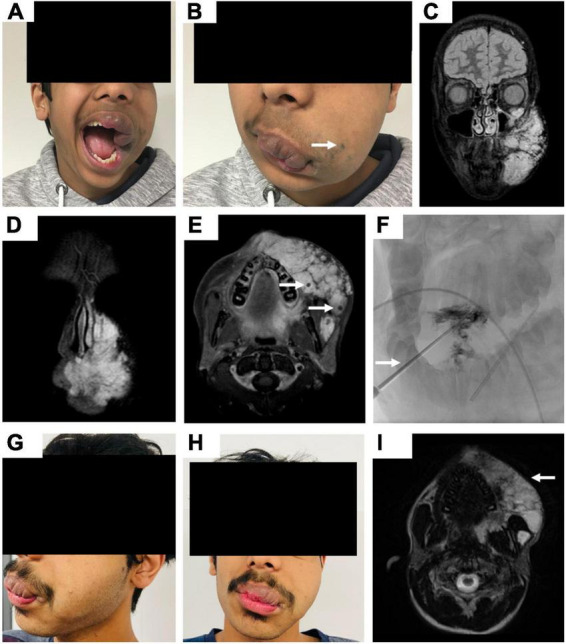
Fourteen-year-old male patient with a venous malformation (VM) primarily affecting the nasal, buccal, mental, and labial area of the left face. (A,B) Clinical photographs demonstrate the appearance of the patient with extensive swelling in the areas affected by the VM and enormous labial deformation on the left side. Superficial bluish discoloration of the skin is marked by an arrow. (C–E) Coronar and axial fat-saturated T2-weighted MR images present typical hyperintense signal of the facial VM with multiple hypointense spots representing phleboliths (arrows). (F) Periprocedural digital subtraction angiography (DSA) images while successful percutaneous sclerotherapy of oropharyngeal compartments, the arrow marks the oral inserted angiocatheter. (G,H) Clinical photographs show the appearance of the patient 3 years post sclerotherapy with reduced swelling of the affected areas. The patients’ lip closure can be performed completely as well as no functional problems with food intake occur. (I) In parallel, post-treatment axial T2-weighted MR image reveals a reduced volume of the VM (arrow).
